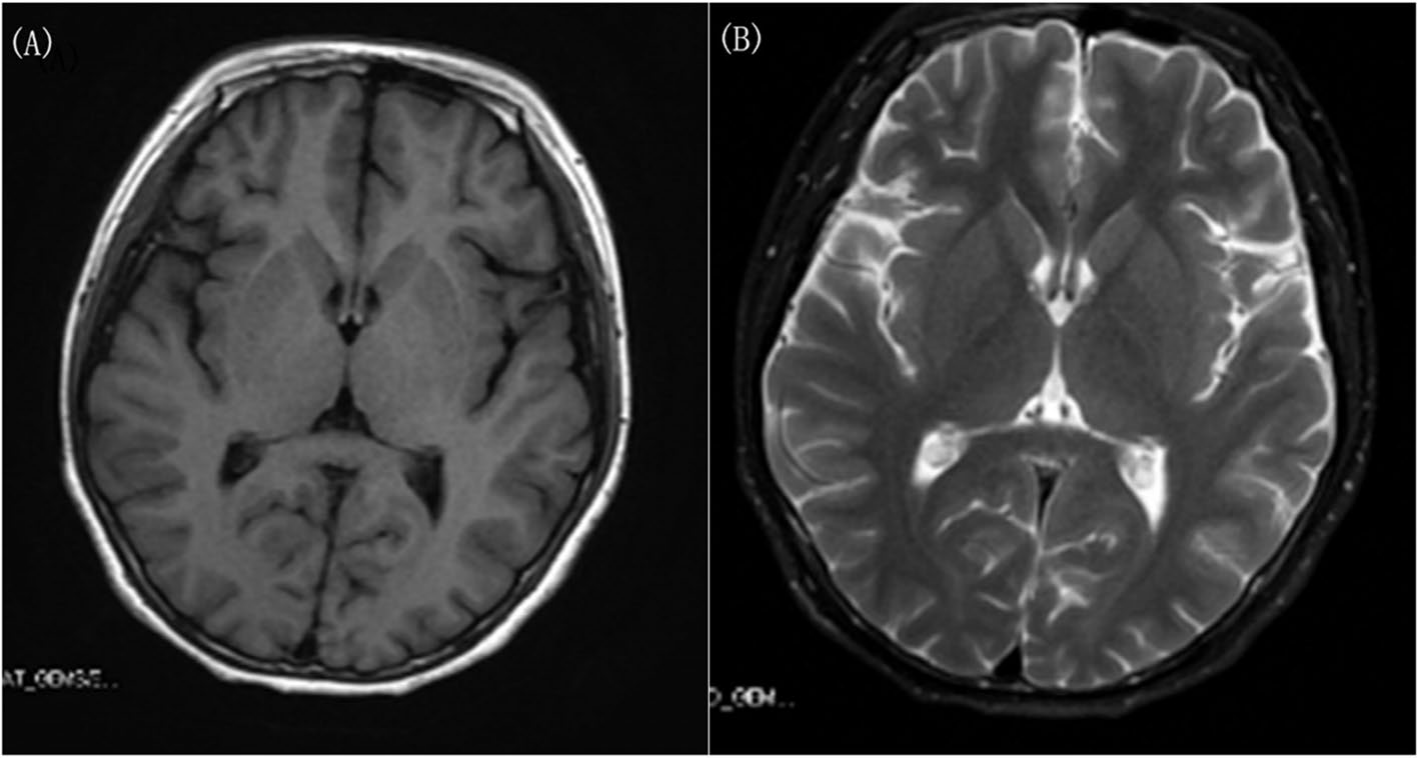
Brain magnetic resonance imaging in July 2020. (a) No positive results were found in the T1 sequence (b) no positive results were found in the T2 sequence
